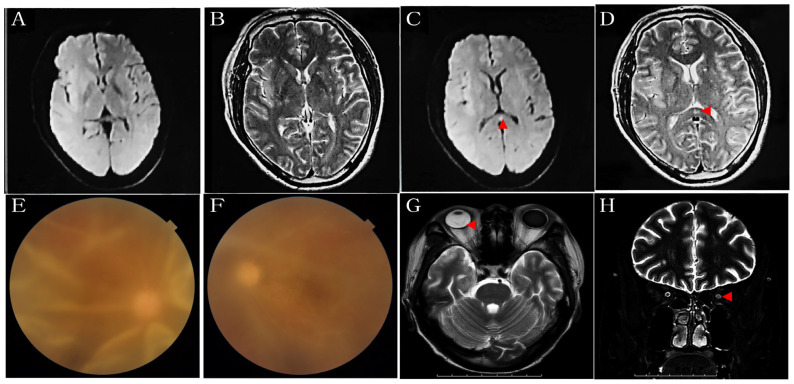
MRI findings of Case 13. Brain MRI of Case 13 during the acute stage of encephalitis showed DWI and T2 hyperintense lesions in the right temporal lobe (A,B) and splenial corpus callosum (arrowheaded) (C,D). Fundus photography of both eyes showed retinal necrosis and detachment (E,F). MRI showed “V “shaped detachment of his right retina (arrowheaded) (G) and signal increased in the left optic nerve (arrowheaded) (H).
